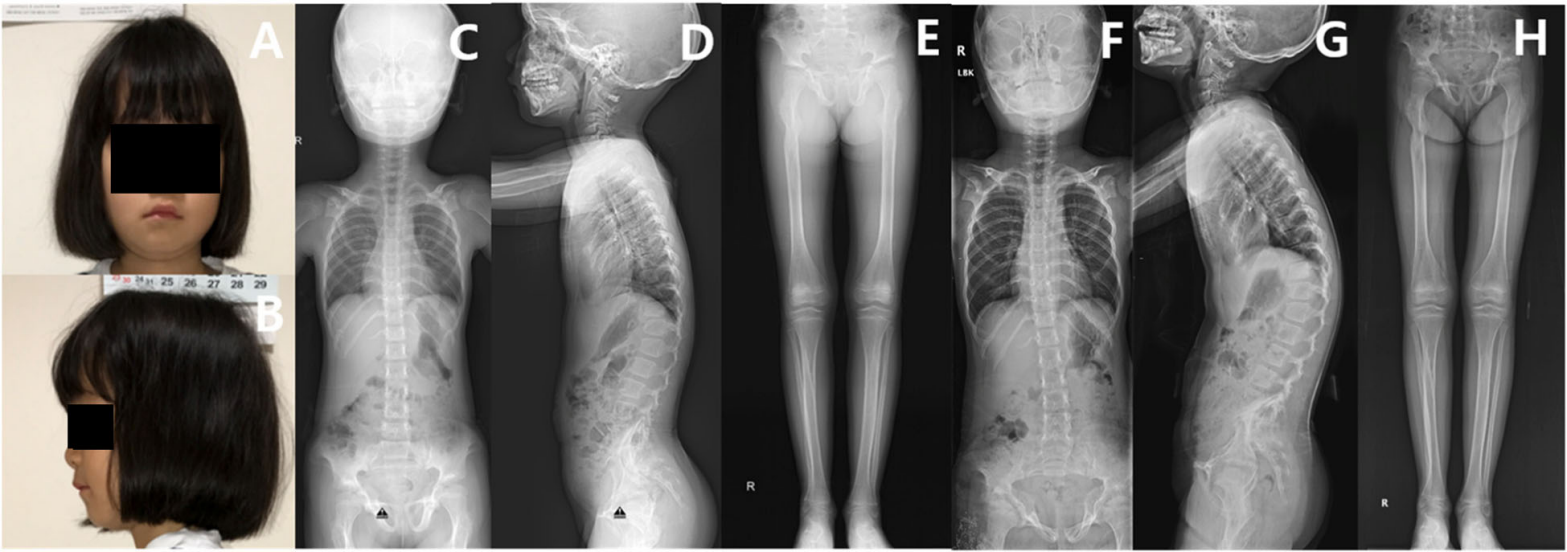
Photographs and plain radiographs of the patient’s older sister. a, b The patient’s older sister had a nearly normal-appearing face. c, d At 6 years of age, plain radiographs revealed normal spine alignment. e However, bilateral coxa valga and Erlenmeyer flask deformities were noted at 6 years of age. f, g At age 9 years, thoracolumbar kyphoscoliosis was apparent. h The coxa valga and Erlenmeyer flask deformities were still present
